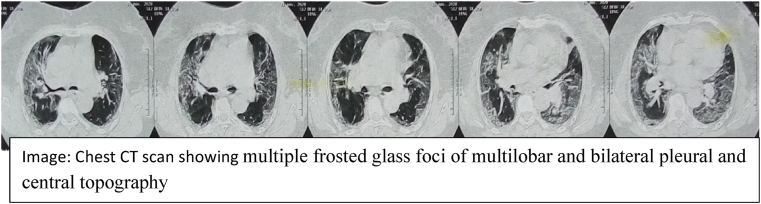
Chest CT scan showing multiple frosted glass foci of multilobar and bilateral pleural and central topography.
